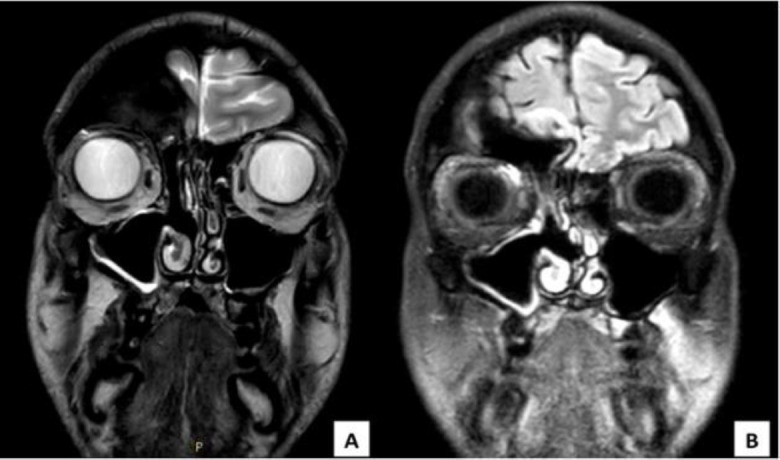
Coronal view of 10 months MRI follow-up weighted in T2 (A) and T1 with gadolinium (B). The right frontal and maxillary sinuses appear completely aerated. The previous area of the mucopyocele is indicated with a black arrow
